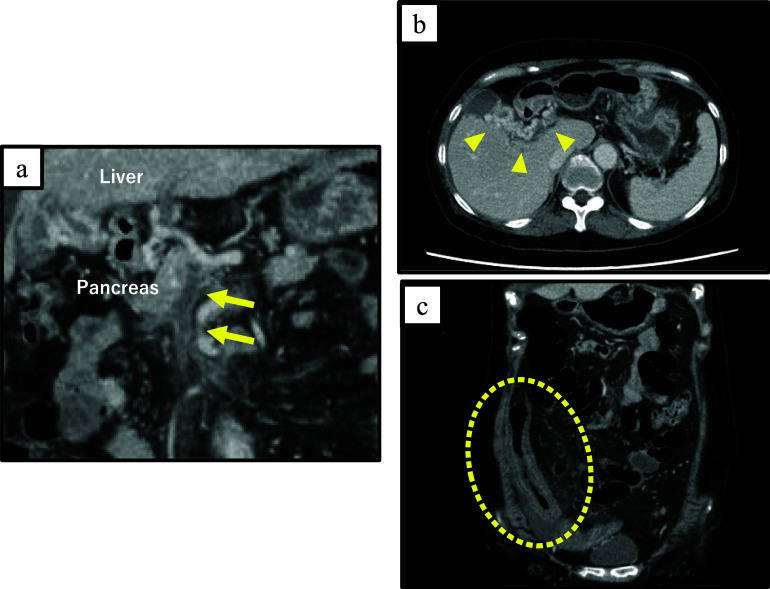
Contrast-enhanced computed tomography. a Portal vein thrombosis and mesenteric vein thrombosis from the superior mesenteric vein (arrow). b Cavernous transformation at the porta hepatis (arrowheads). c Poor contrast area of the small bowel loops (dotted line)
